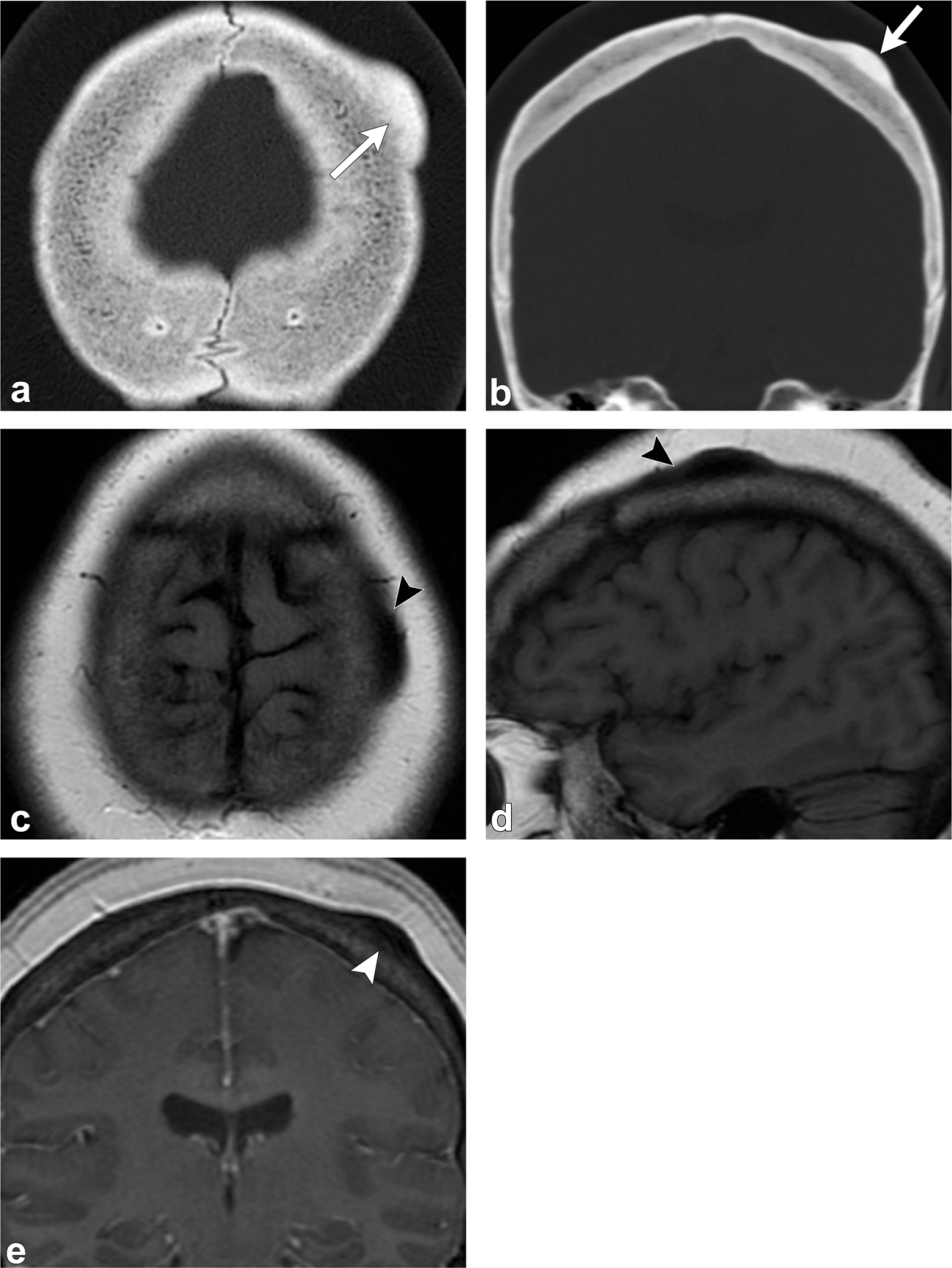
Osteoma. Axial (a) and coronal (b) head CT images show a juxta-cortical lesion along the outer table of the left frontal bone (*arrows*). Axial T1-weighted (c), sagittal T1-weighted (d) and coronal post-contrast T1-weighted (e) images depict the sclerotic/osteoblastic nature of this lesion (*arrowheads*). Note the well-defined margins and lack of enhancement
